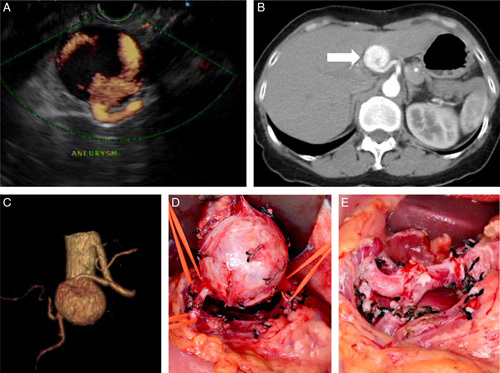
Common hepatic artery true aneurysm (A) Endoscopic ultrasonography showed a common hepatic artery aneurysm (3 cm in diameter). (B, C) The CT scan and CT angiography revealed an aneurysm located between the junction of the common hepatic artery, gastroduodenal artery, and proper hepatic artery. (D) The three feeding vessels of the aneurysm were dissected and controlled individually. (E) After excising the aneurysm, we did end-to-end vascular reconstruction between the common hepatic artery and the common orifice of the proper hepatic artery and the gastroduodenal artery.
